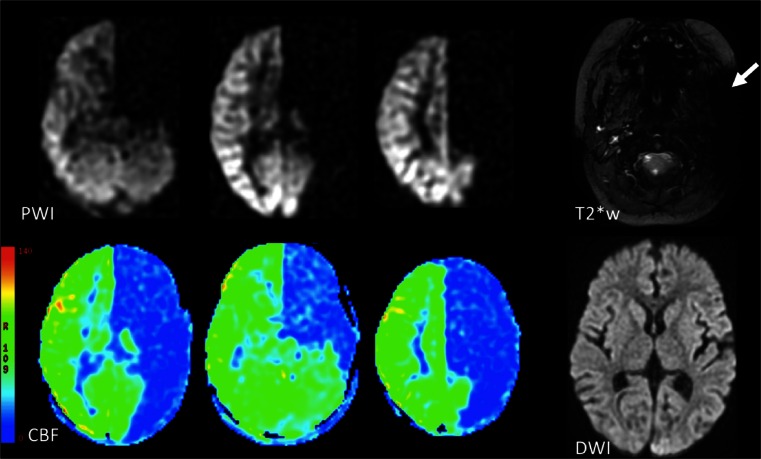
Labeling failure in the left internal carotid artery. There is absence of signal on the ASL perfusion-weighted images (*PWI*) and corresponding cerebral blood flow (*CBF*) maps in the arterial territory of the left internal carotid artery. Note the large susceptibility artefact in the area of the left internal carotid area on the T2* weighted (*T2*w*) image, as the likely cause of the labeling failure. Diffusion weighted imaging (*DWI*) is normal, supporting interpretation of this finding as an artefact
